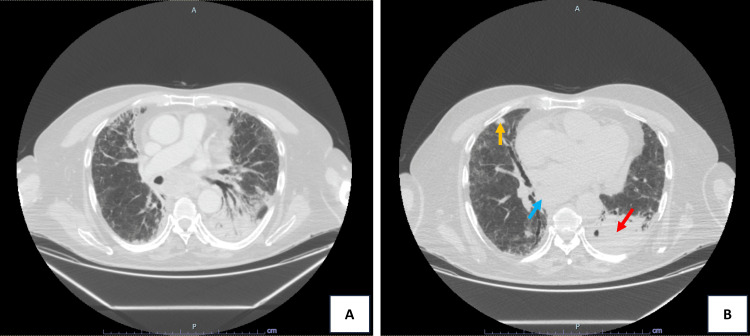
Axial chest CT scans performed for oncologic re-staging (seven months after immune checkpoint inhibitor discontinuation and 10 months after radiotherapy), demonstrating oncological disease progression. (A) and (B) show enlarging mediastinal nodal disease (blue arrow), growth of the primary tumor in the left lower lobe (red arrow) with collapse of the underlying parenchyma, and new multiple bilateral subpleural and pulmonary nodules compatible with metastases (yellow arrow). Motion artifacts limited the assessment of the lung parenchyma; however, there was no evidence of fibrosis progression.
